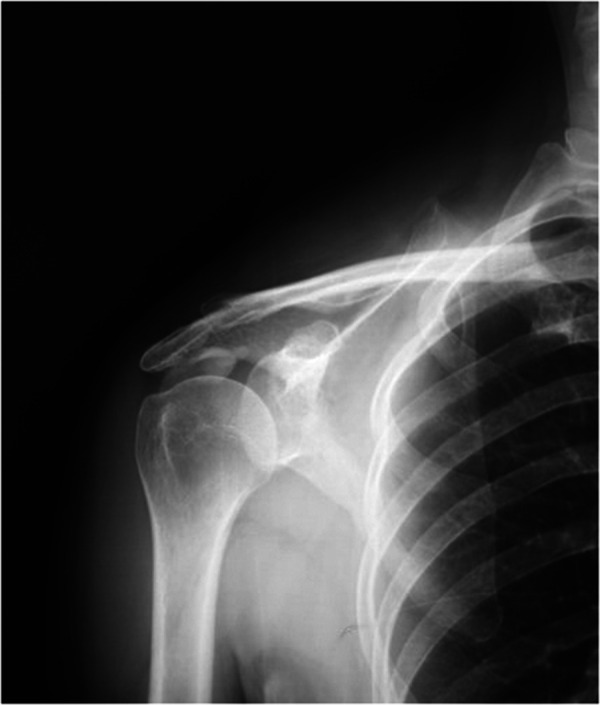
X-ray (front) showed clear calcific deposits in the target shoulder.
